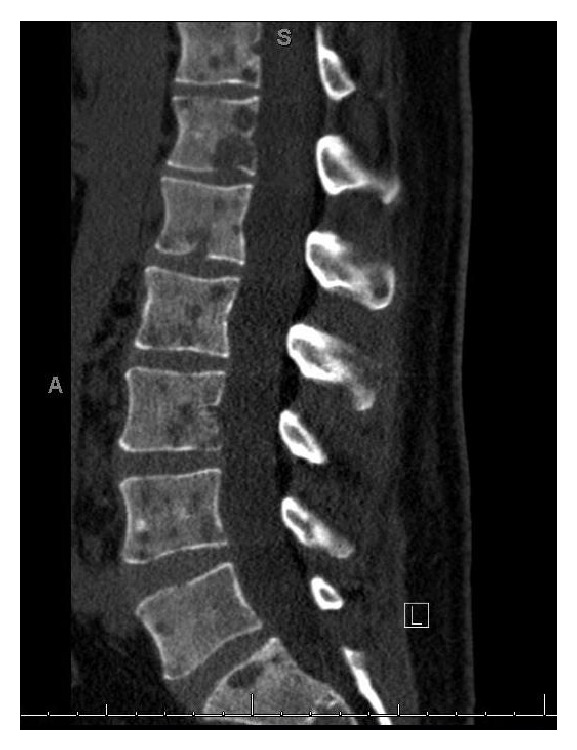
CT lumbar spine without contrast showing innumerable lytic lesions seen throughout the lumbar spine.
